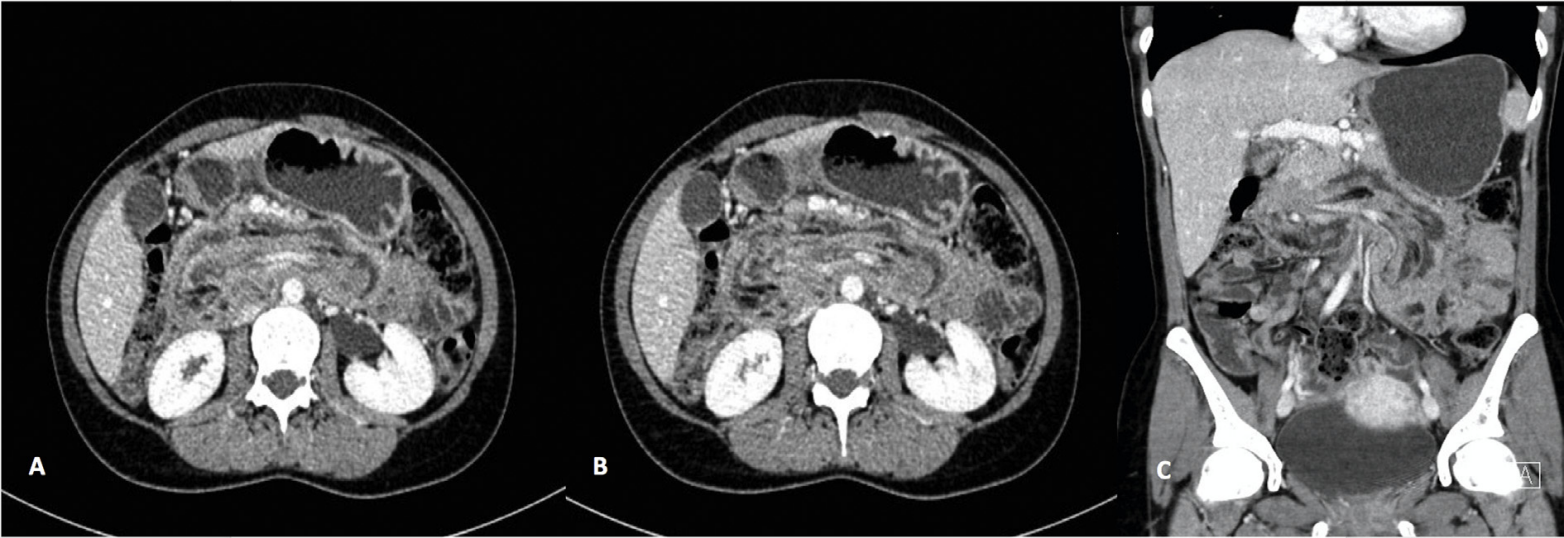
A and B: CT scan of abdomen and pelvis with contrast axial views reveals a significantly dilated stomach with no detectable gastric lesion. The third part of the duodenum is distended by a sausage shaped intraluminal structure with alternating fat planes and dilated vessels reaching up to the first part of the duodenum in keeping with retrograde jejuno-duodenal intussusception. There are no detectable soft tissue lesions and the rest of the bowels are unremarkable. C: Coronal view with the same findings.
